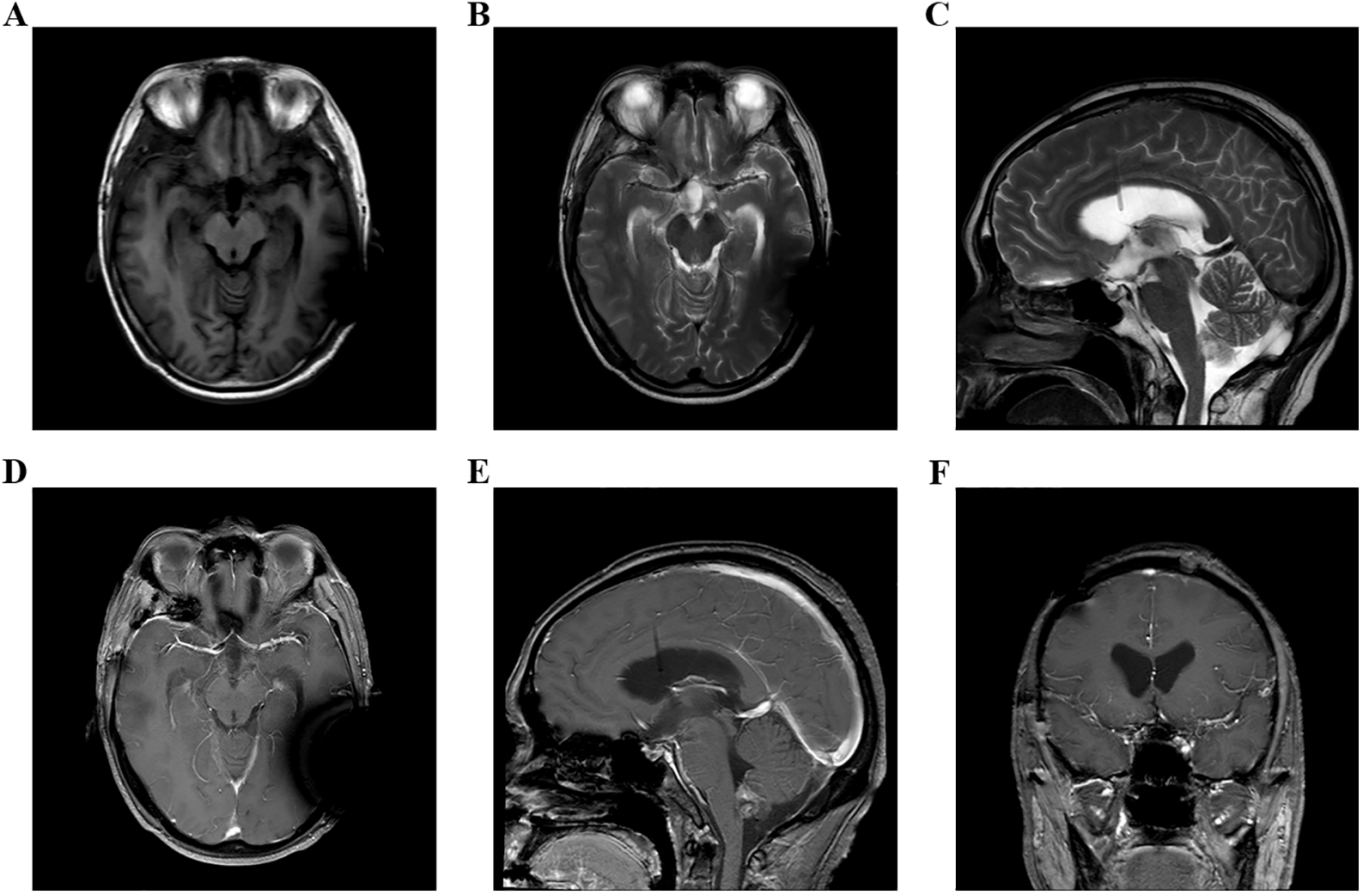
(A–F) Head MRI examination on December 9, 2023, indicated that the volume of third ventricle and bilateral lateral ventricles were smaller than before.
